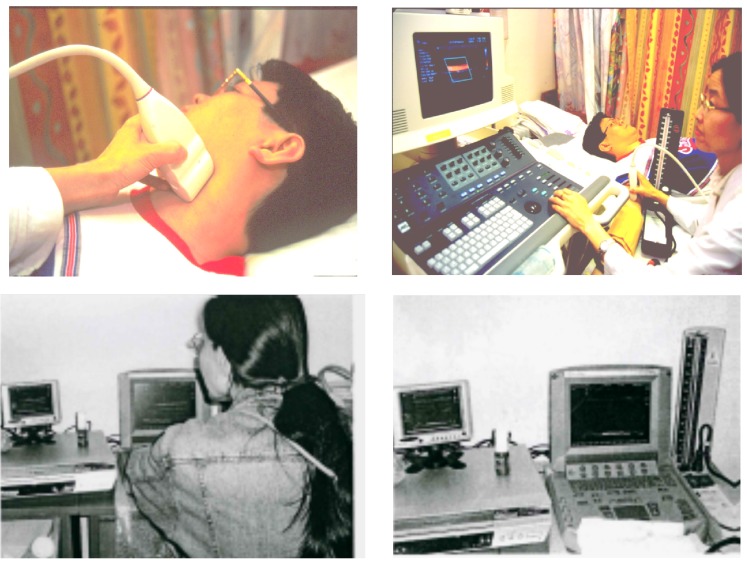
Ultrasound scan of carotid artery for carotid intima-media thickness and brachial endothelium-dependent dilation (endothelial function), using an ultrasound console (above); or a portable ultrasound machine at field work in northern China (below).
